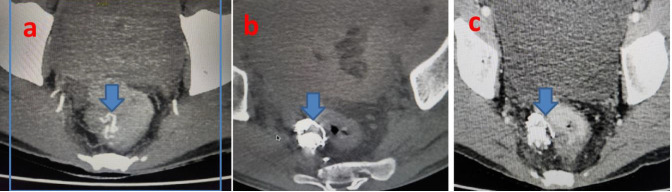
CT Guided percutaneous glue injection of rectal varix: pre-procedure axial CT angiogram of pelvis rectal varix. The CT
